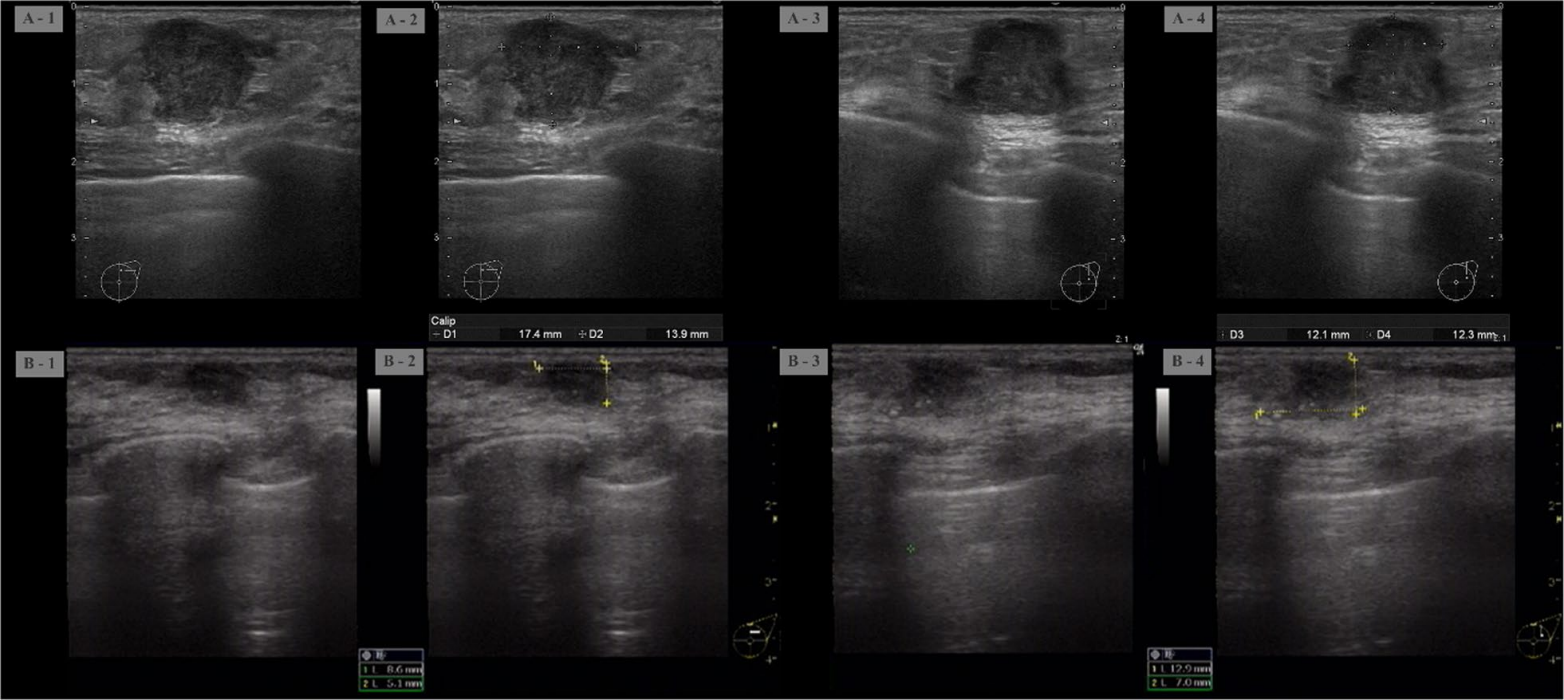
Ultrasound findings pre- and post-trastuzumab therapy. A At the time of diagnosis. B After a single dose of trastuzumab monotherapy. Tumor diameter appeared to have shrunk overall
